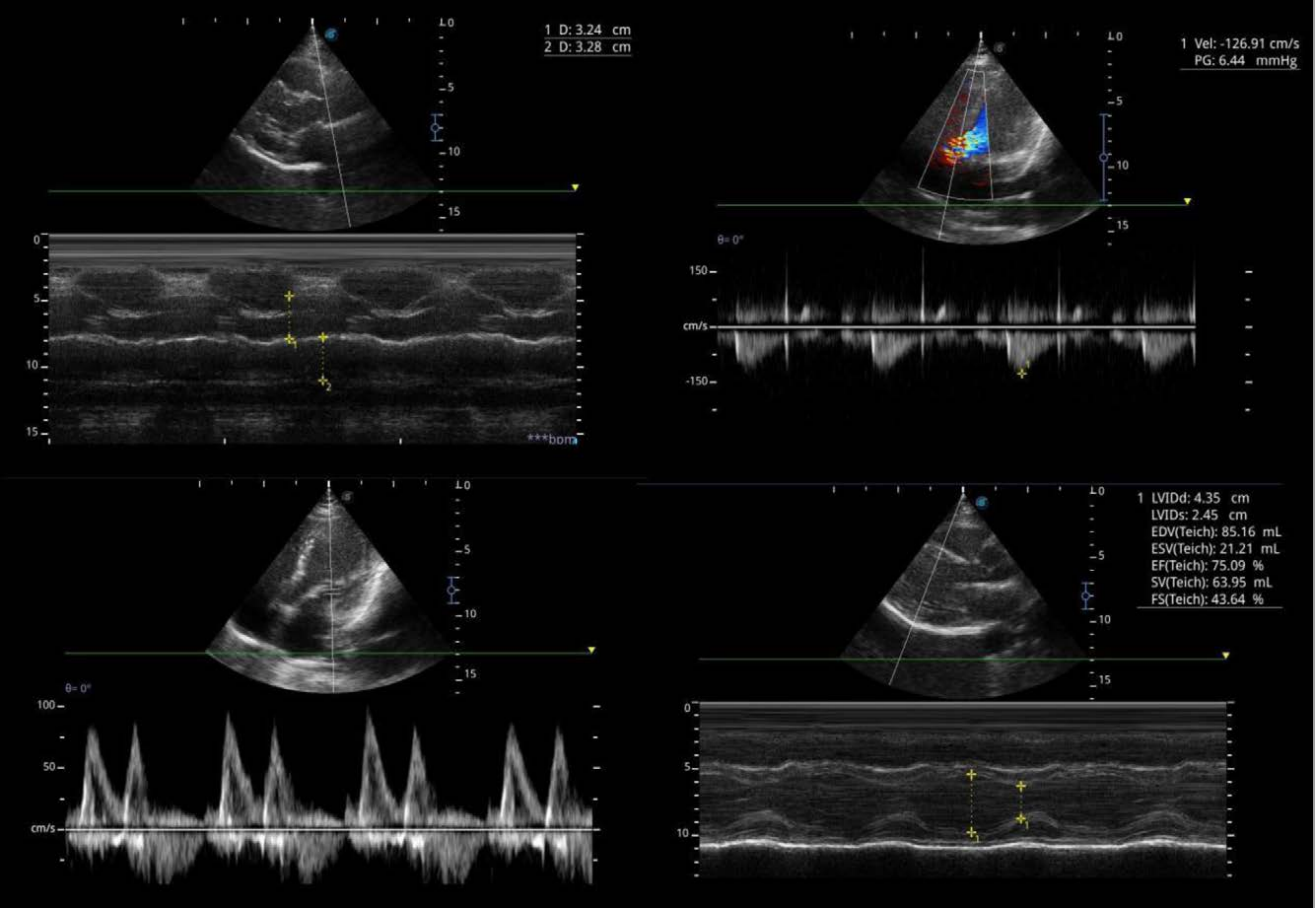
Baseline echocardiogram showing no structural or functional abnormalities.
